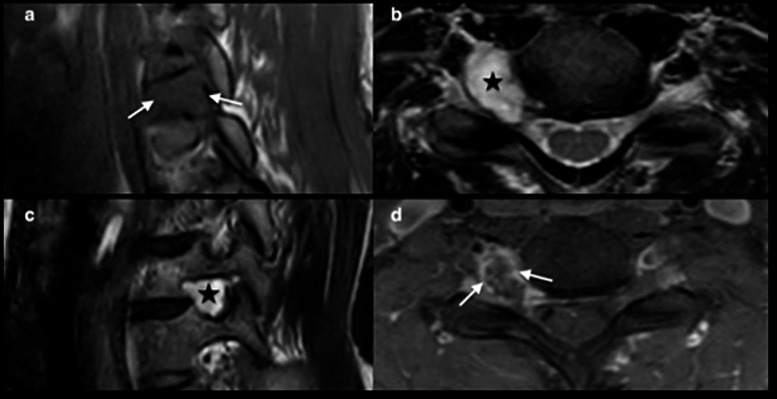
Pre-operative cervical MRI. Panel a: Sagittal T1-weighted images; Panel b: Axial T2-weighted images; Panel c: Coronal oblique T2-weighted image; Panel d: Axial T1 Fat sat weighted image after contrast injection
